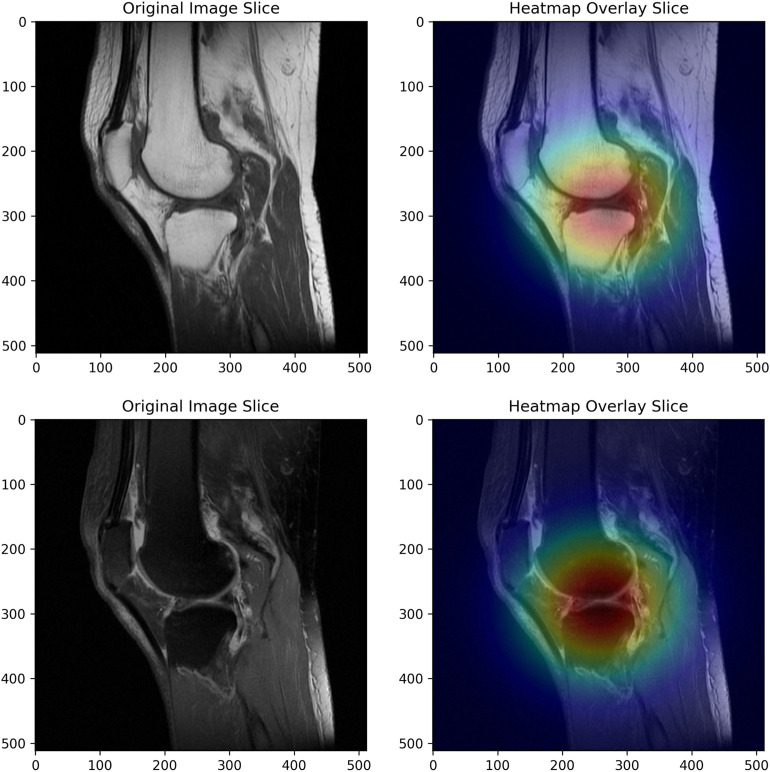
The learned feature maps of MobileNetV2 for MRI images.
